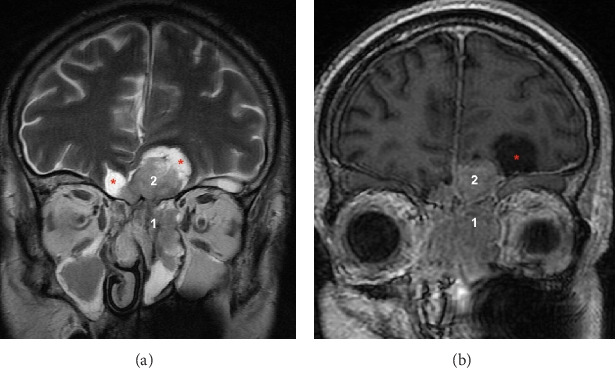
Brain MRI at diagnosis in T2 fast spin-echo (a) and T1 (b) weighted sequences in coronal sections. (1) Intranasal part of the tumor. (2) Intracranial part of the tumor. Red asterisks: peritumoral cysts. This imaging reveals a large, mixed solid and cystic tumor mass, heterogeneous on T2-weighted sequences and isointense on T1, with contrast enhancement. The lesion is both intra- and extracranial, extending across the cribriform plate. Intracranially, it involves the bilateral frontal regions. Extracranially, it invades the nasal cavities. There is evidence of osteolysis of the cribriform plate.
